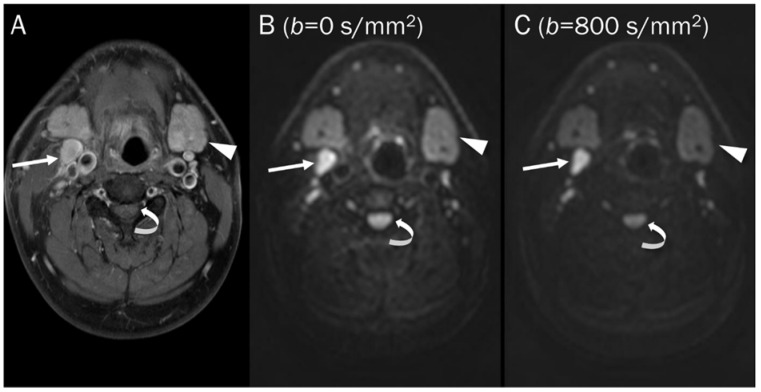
DWI of a pathologic lymph node, a normal submandibular gland, and a normal spinal cord. (A) Axial T1-weighted enhanced image of the neck shows a pathologic lymph node (arrow), a normal submandibular gland (arrowhead), and a normal spinal cord (curved arrow). These hypercellular tissues remain bright on DWI with increasing *b*-value images (B,C).
